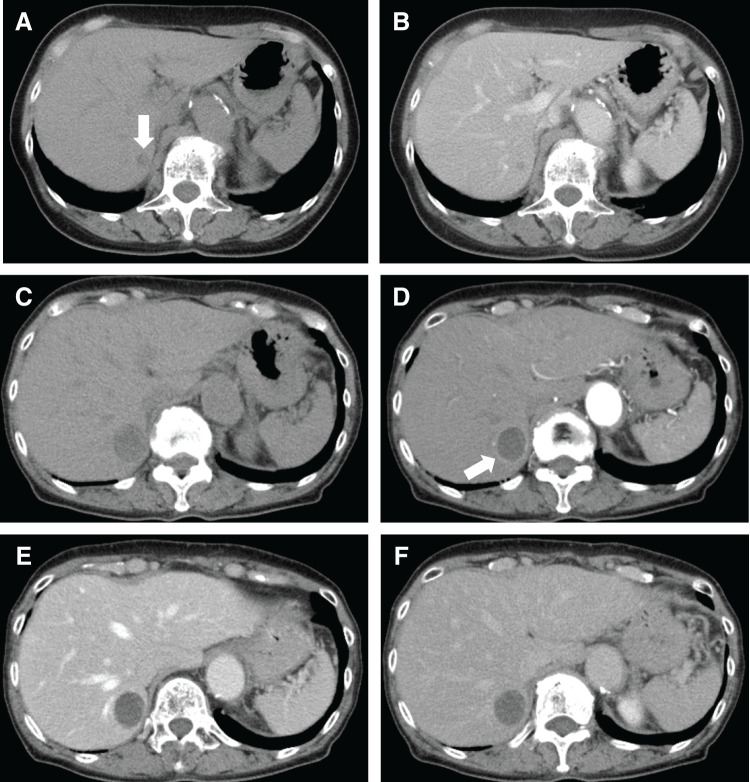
Abdominal CT findings. CT findings 1 year prior to the visit to our facility revealed a small low-attenuation nodule measuring 6 mm in diameter (arrow) (A, non-contrast and B, contrast-enhanced). (C) A non-contrast CT scan before surgery revealed a low-attenuation nodule measuring 20 mm in diameter. A contrast-enhanced CT scan showed a ring-like contrast enhancement with an oligemic interior (arrow) (D, arterial phase, E, portal phase, and F, late phase).
